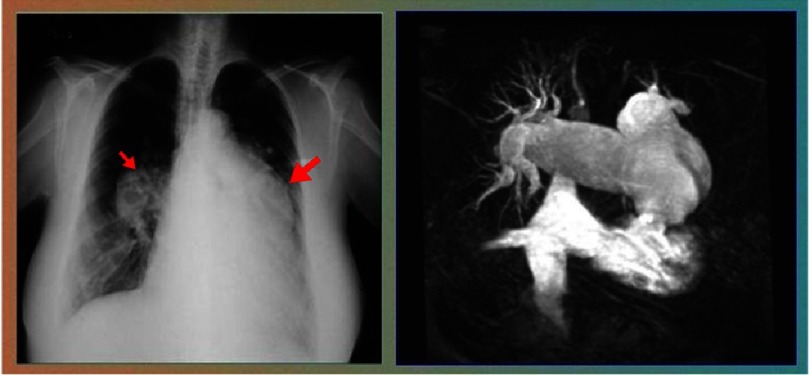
A chest radiograph of a 52-year old man with pulmonary hypertension due to schistosomiasis. The X ray to the left and right show the dilatation of left and right pulmonary arteries (arrows). the right panel showing the characteristic dilation of both main right and left pulmonary artery in patients with schistosomiasis pulmonary hypertension (images: courtesy of Dr Angela Bandeira).
